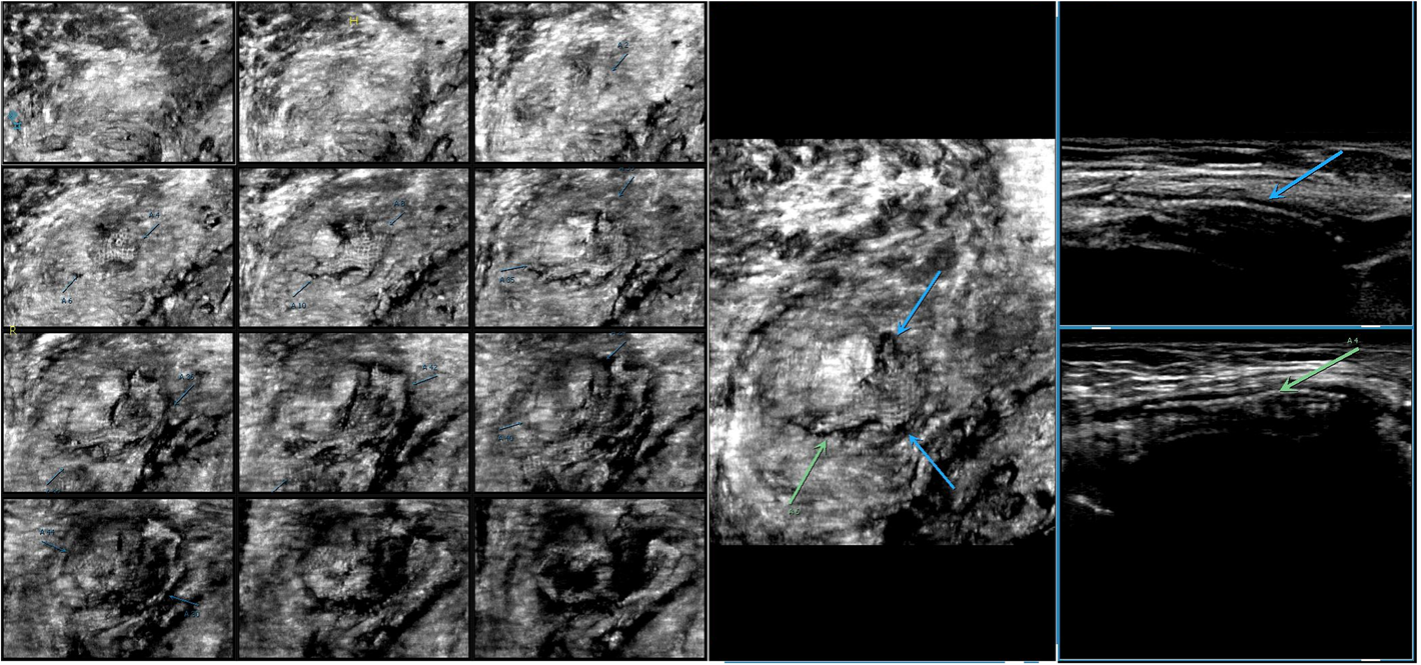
The shrinking mesh showed in the ultrasonography. The shrinking mesh was showed on coronal consecutive image and 3D display. Arrows referred to the shrinking mesh
